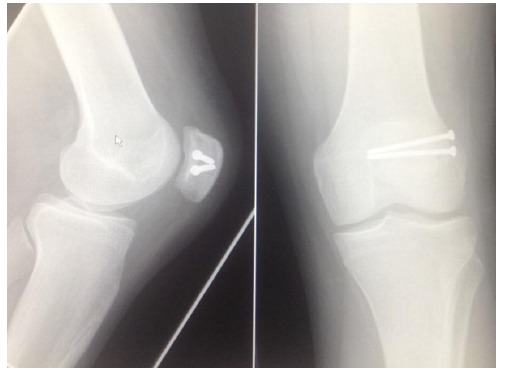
Post-operative radiographs taken at 6 weeks.
